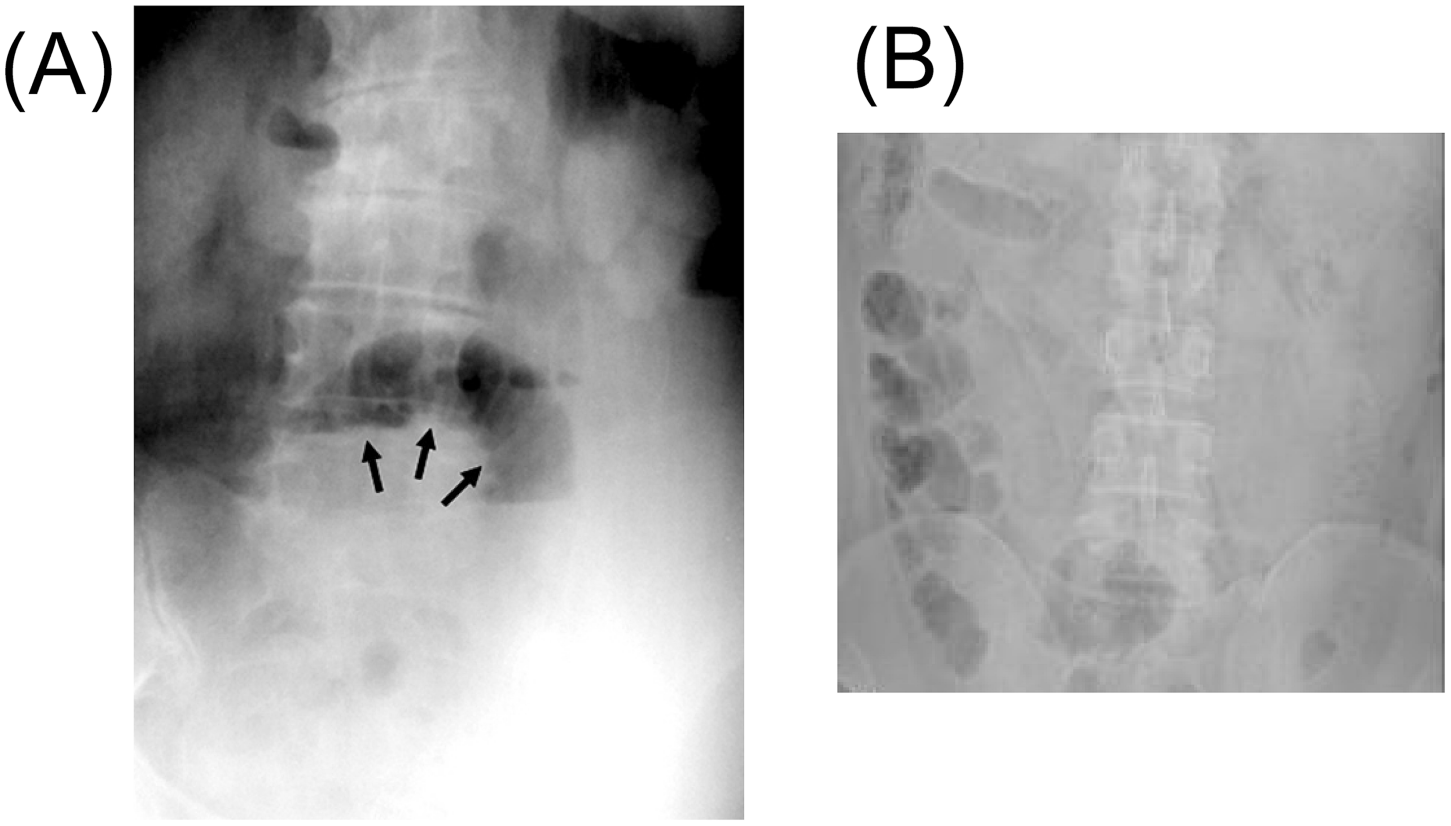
The figure shows the X-ray after intestinal perforation caused by a fishbone. (A) The supine abdominal plain film shows dilated small bowel loops, suggesting the possible presence of small bowel obstruction. There is no obvious radiopaque foreign body (black arrow). Adapted from reference (50) with permission from Galenos. (B) The abdominal X-ray shows the formation of a localized central intestinal obstruction. Adapted from reference (139) with permission from Springer Nature.
